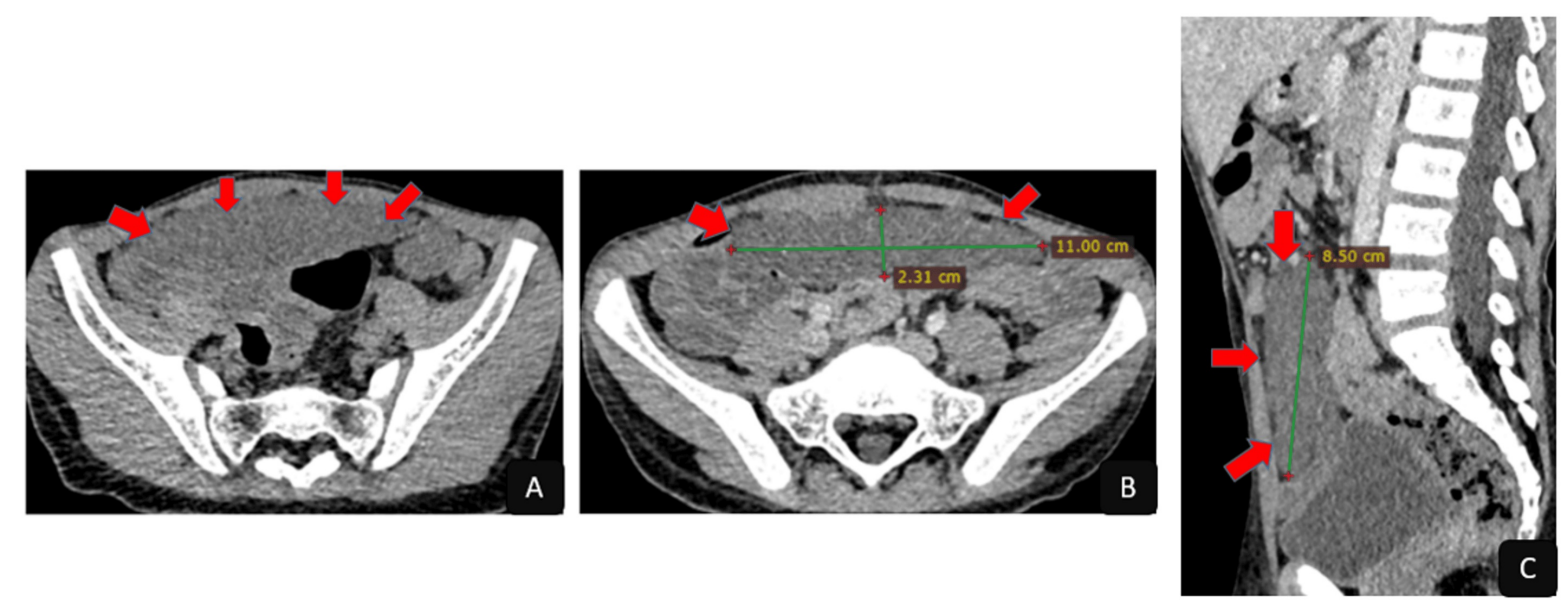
Abdominopelvic CT scan (A) Axial slice before contrast administration; (B) axial slice after contrast administration; (C) sagittal reconstruction. Well-defined, spontaneously hypodense anterior mesenteric mass, with no enhancement following contrast administration.
